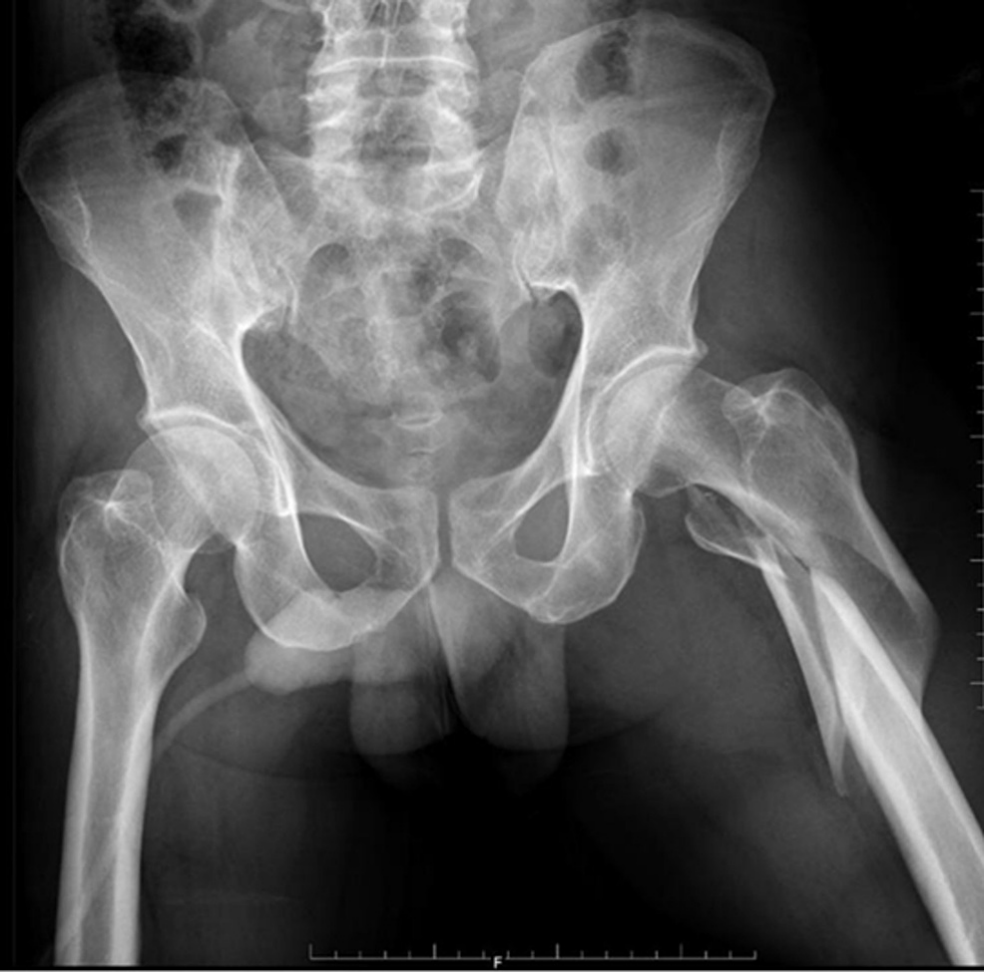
Pre-operative pelvis with bilateral hip - AP radiograph AP: anteroposterior
